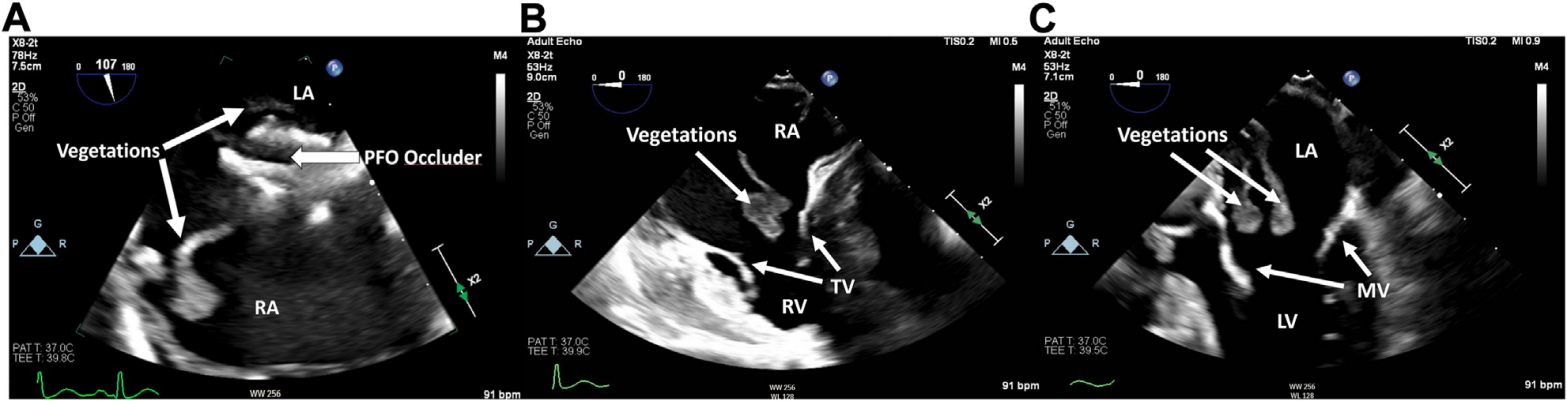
Two-dimensional transesophageal echocardiogram, midesophageal (A) bicaval, systolic view (107°), (B) modified, 4-chamber diastolic view (0°), and (C) 5-chamber diastolic view (0°), demonstrates the PFO occluder with large biatrial vegetations (A), vegetations within the RA, but sparing the TV (B) and vegetations within the LA, but sparing the MV (C). *LA*, Left atrium; *LV*, left ventricle; *MV*, mitral valve; *RA*, right atrium; *RV*, right ventricle; *TV*, tricuspid valve.
